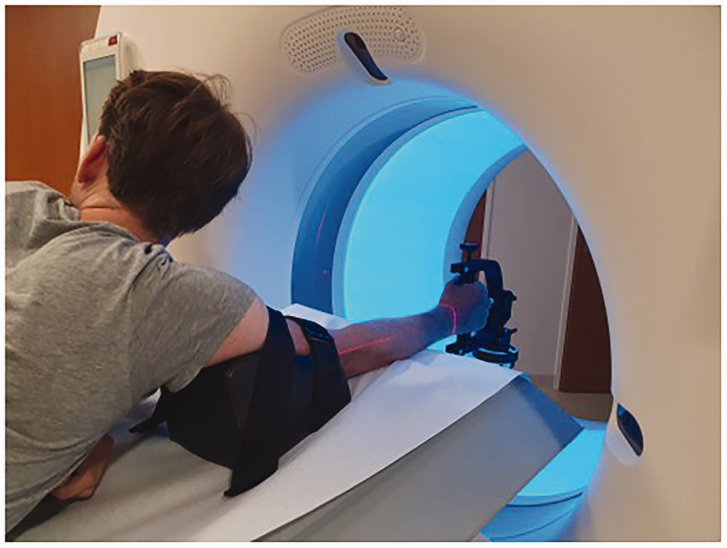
Patient positioning and hand guiding device for forearm rotation during 4-D CT.
